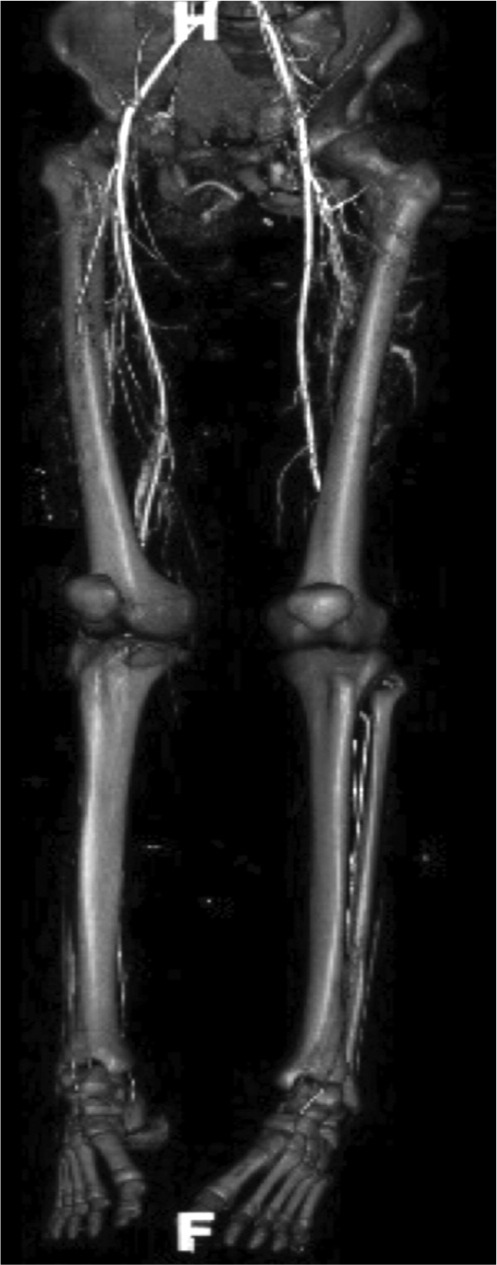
3D reconstruction Computed Tomography (CT Imaging) revealed blood collection over left periacetabular region and thrombosed of right popliteal artery with good distal run-off.
